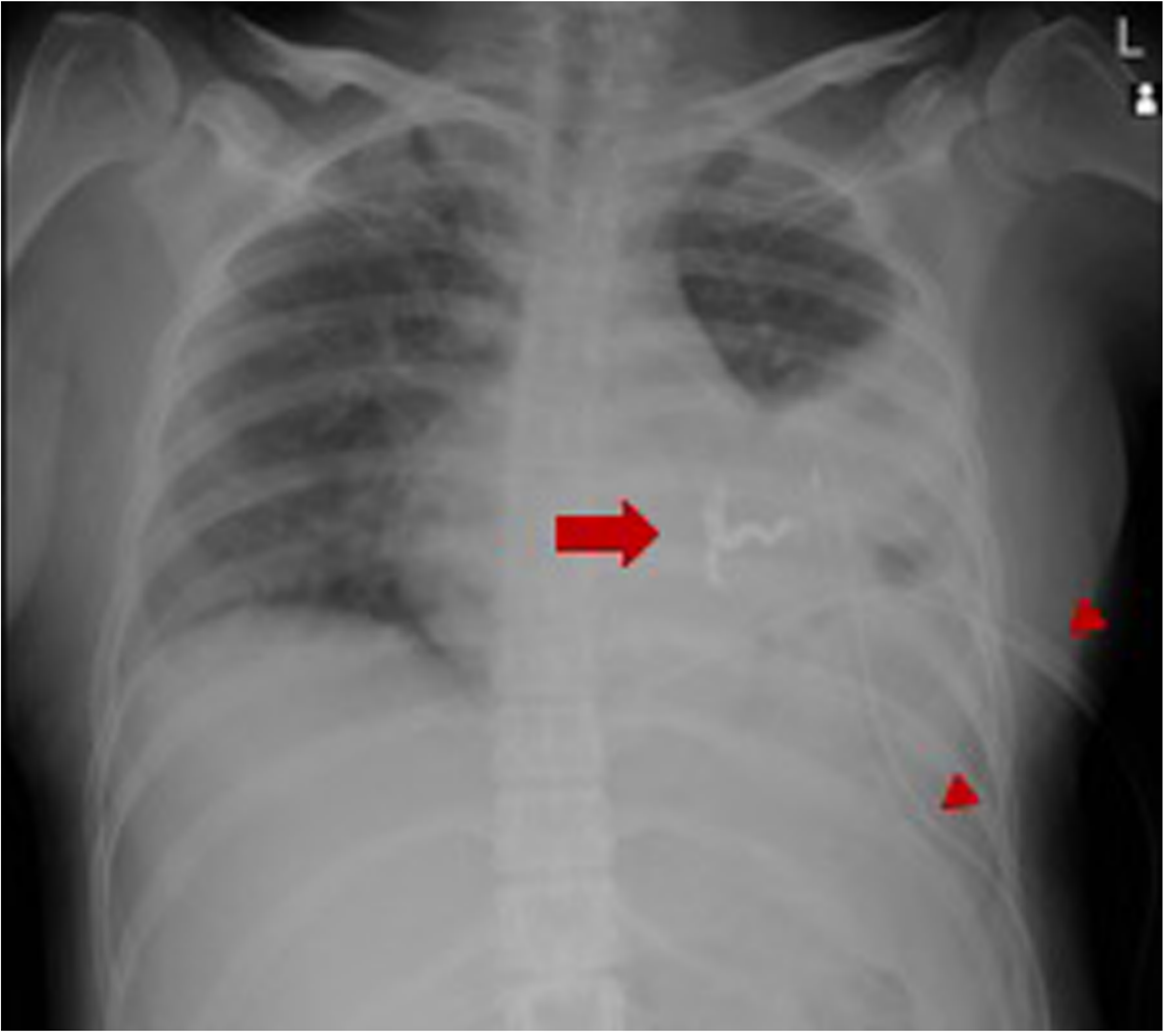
Chest X-ray imaging at 30 weeks of gestation. Embolization coils (the arrow) and chest tubes (arrowheads) are placed for the pleural hemothorax treatment due to the ruptured arteriovenous malformation
